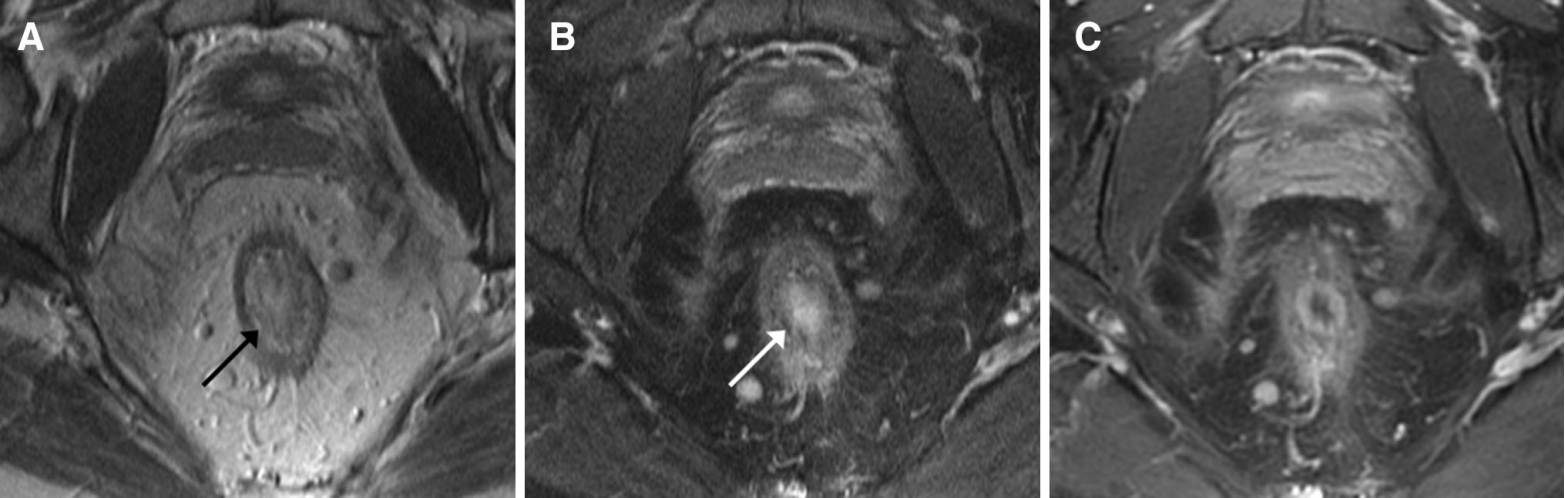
A 53-year-old female with Crohn’s disease and ulcerative proctitis at endoscopy. A Axial oblique T2-weighted image shows high mural signal intensity and B low signal intensity on axial oblique fat-saturated T2-weighted image corresponding to mural fat (*arrow*). C Axial oblique fat-saturated post-contrast T1-weighted images shows moderate enhancement of the rectal wall and perimural fat tissue. In addition, wall thickening and multiple mesorectal lymph nodes are present
